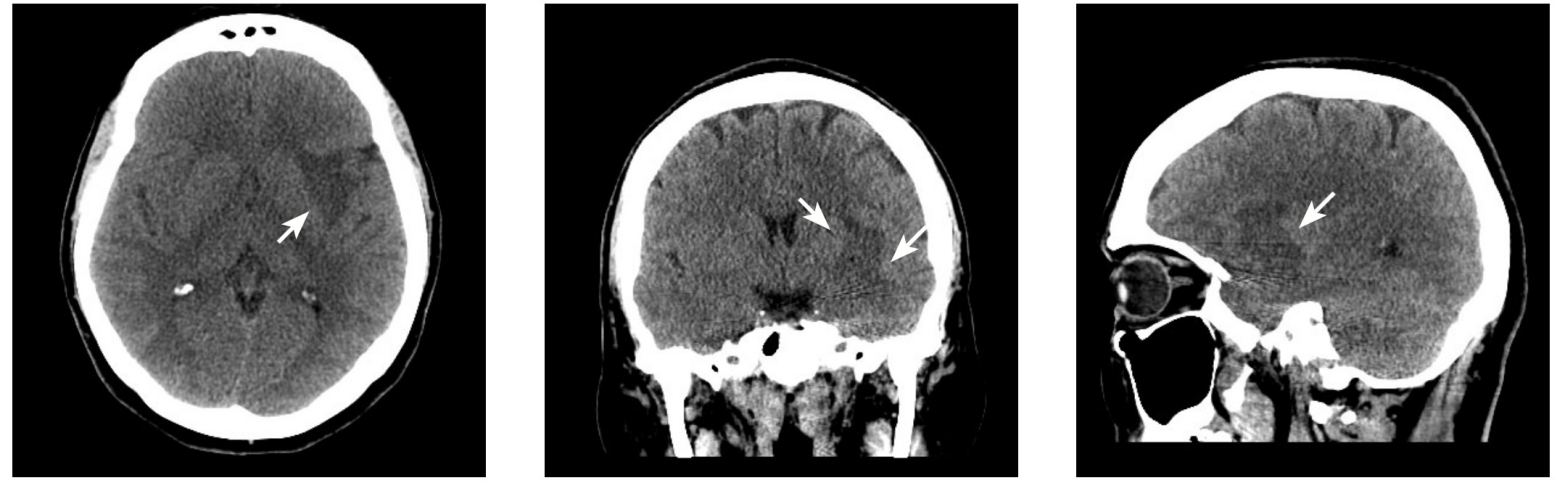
Axial computed tomography indicative of vasogenic edema in the left anterior perisylvian region (arrows).
